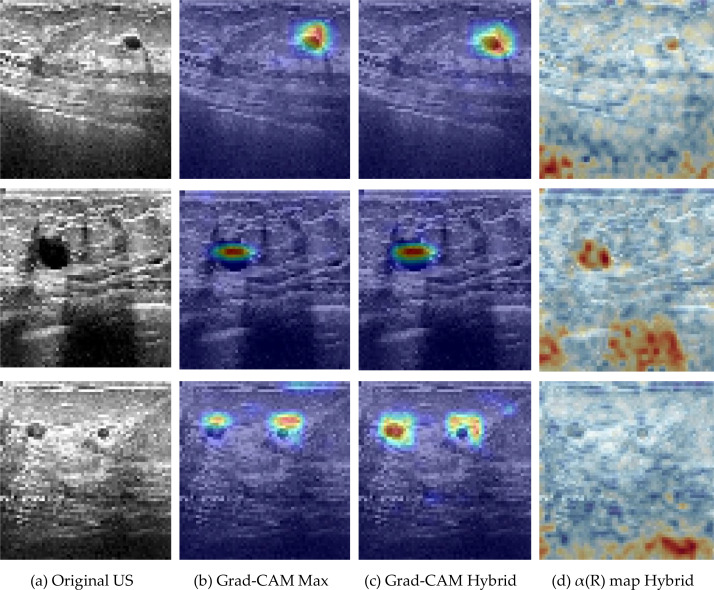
Interpretability analysis comparing Max and Hybrid pooling models on three representative test cases: (a) original ultrasound image, (b) Grad-CAM activation (Max pooling), (c) Grad-CAM activation (Hybrid pooling), and (d) spatial α(R) entropy weight map from the AdvancedLearnableEntropyPooling2D layer. Warm colors (α=>1) denote max-like behavior at lesion regions; cool colors (α=>0) denote average-like behavior over homogeneous tissue.
